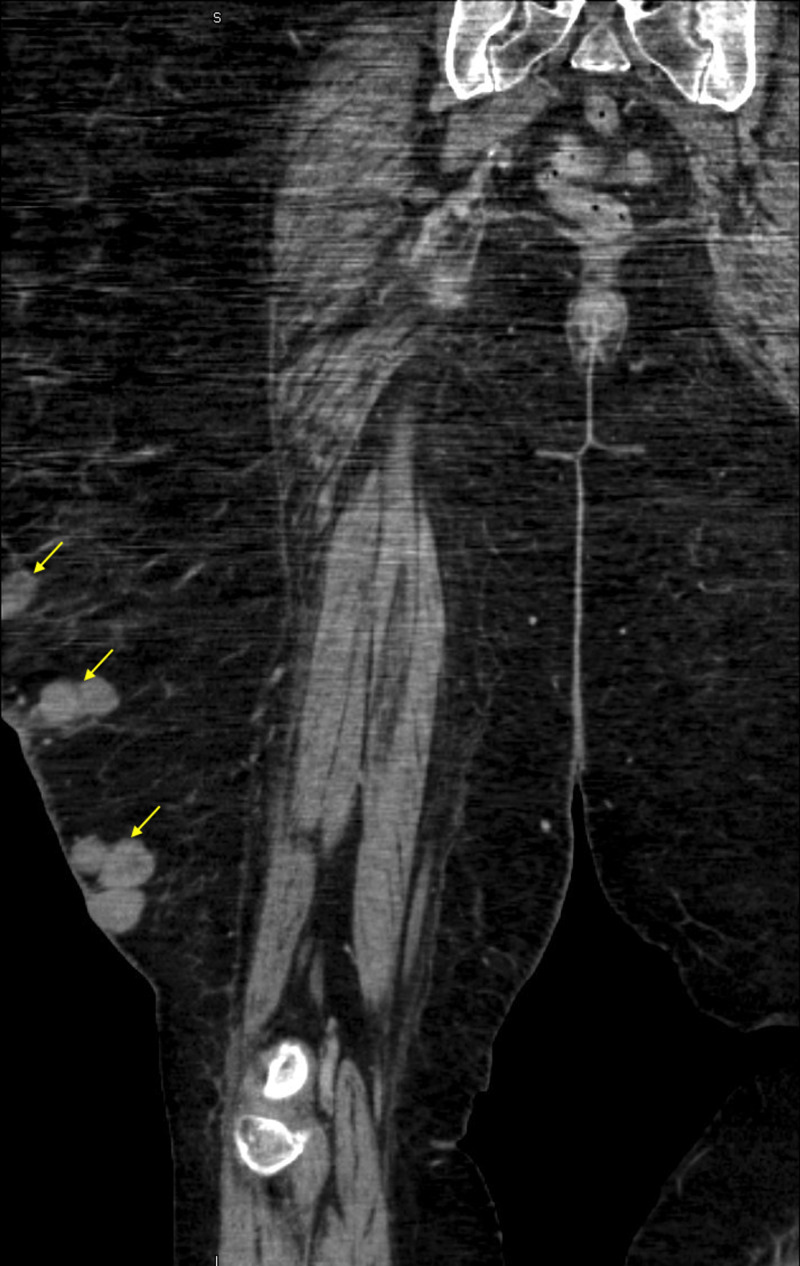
CT Right Lower Extremity with Contrast. Coronal section of the right lower extremity demonstrating multiple conglomerate subcutaneous angiosarcoma lesions. Yellow arrows: Multiple subcutaneous angiosarcomas
